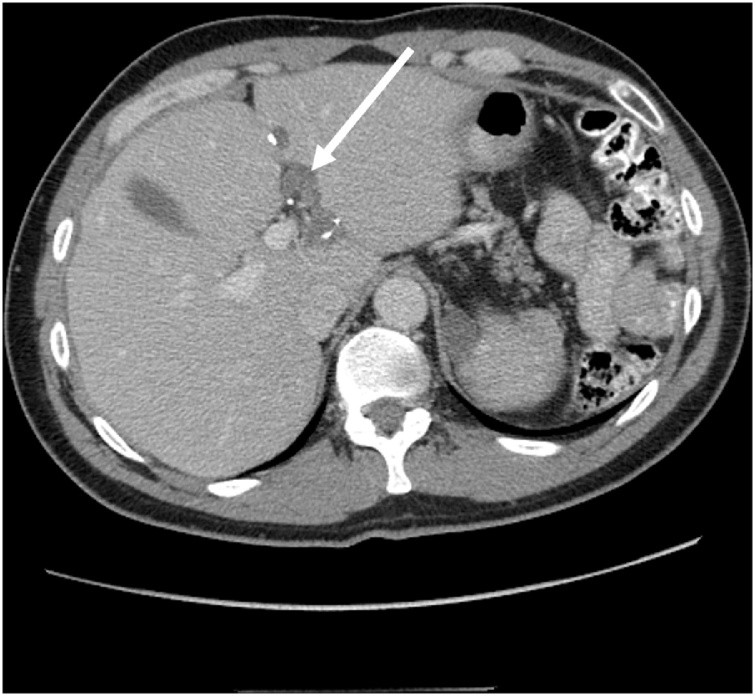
CT cut through the upper abdomen shows cystic collections of mucinous tumor with calcifications along the round ligament. This was compatible with recurrence of pseudomyxoma peritonei beneath a hepatic bridge. This was confirmed at the time of reoperative surgery.
